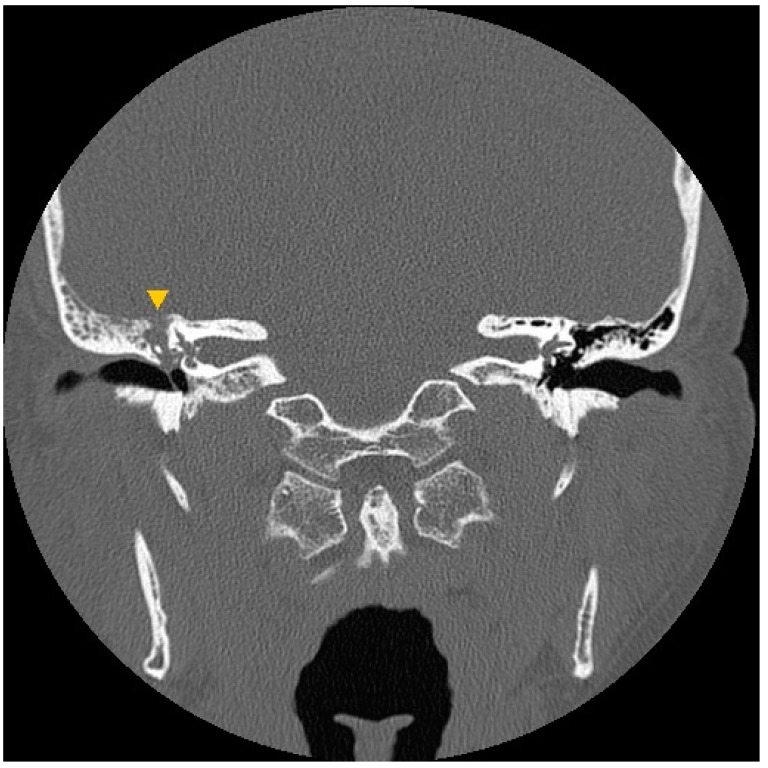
Case 2: A high-resolution computed tomography image of the temporal bone shows obliteration of the right mastoid air cells and middle ear cavity, with erosion of the right tegmen (▼).
